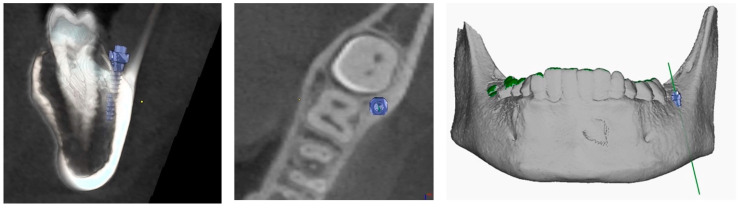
Planning of miniscrew insertion on CBCT (Cone Beam Computed Tomography) from different views.
